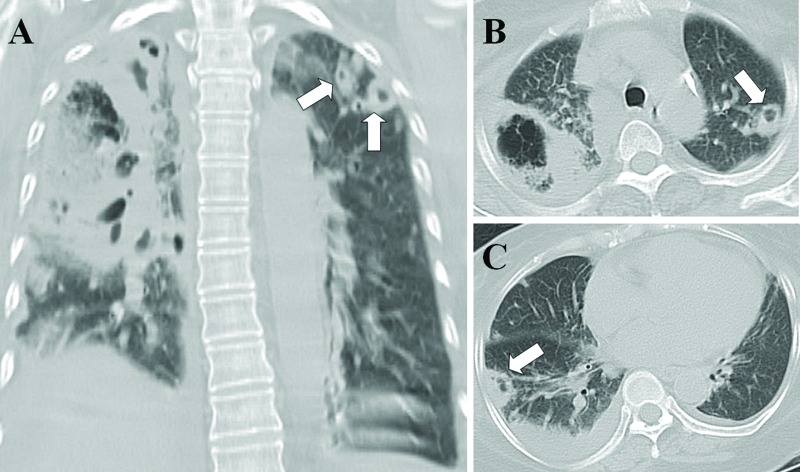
Necrotizing pneumonia with septic pulmonary emboli. (A) A lung window of a coronal-sectional CT scan shows necrotizing pneumonia in the right lung. Multiple different sizes of nodules with cavities in the left upper lobe (arrows), suggestive of septic pulmonary emboli, are observed. (B) A lung window of a cross-sectional CT scan shows necrotizing pneumonia in the right upper lobe and a cavitary nodule in the left upper lobe (arrows). (C) A peripheral wedge-shaped opacity abutting the adjacent pleura in the right lower lobe (arrow) and pleural effusion are seen. The patient was a 62-year-old diabetic woman whose blood and sputum cultures were positive for methicillin-resistant *Staphylococcus aureus*.
